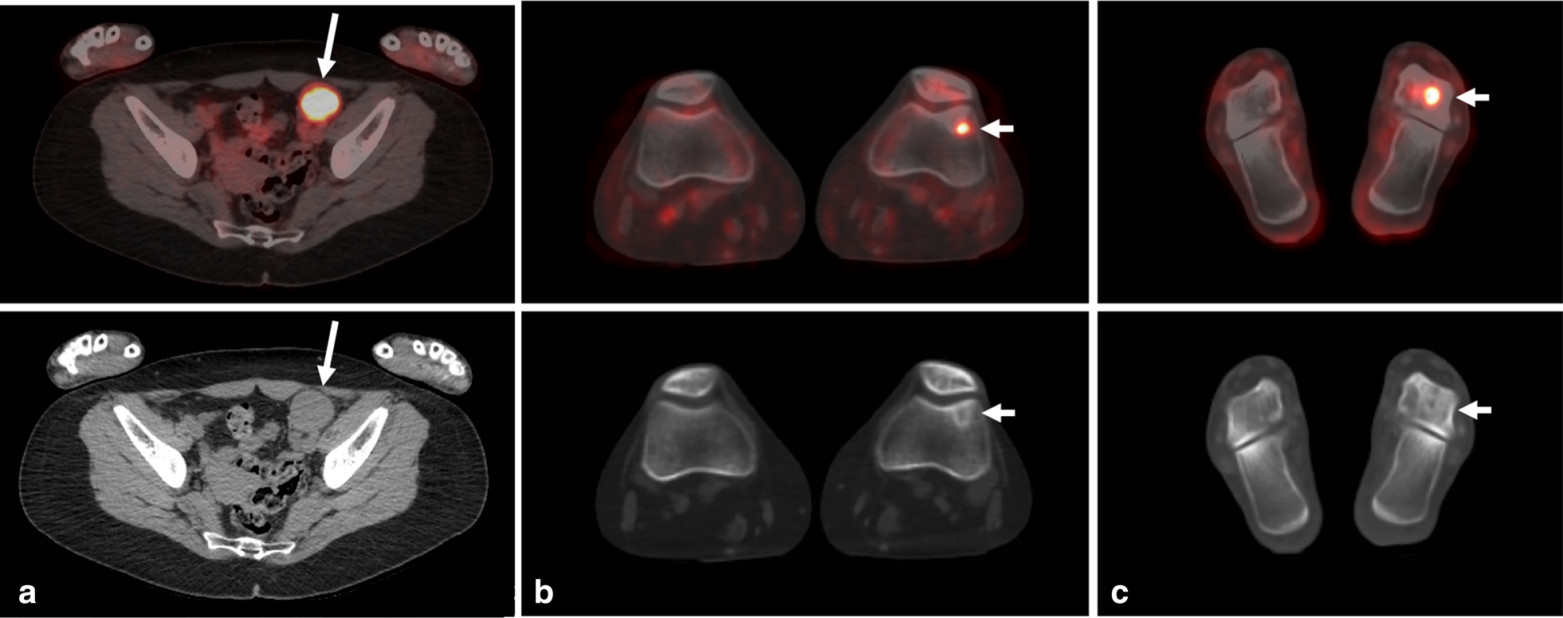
Whole-body ^18^F-FDG PET/CT demonstrates pelvic lymphadenopathy and osseous lesions. a Intense uptake in a 3.8 × 3.6 cm left pelvic side wall lymph node on the fused PET/CT axial image (SUV_max_ 9.8, white arrow). b Moderate FDG uptake in the left lateral femoral condyle (SUV_max_ 3.1, white arrow), on the fused PET/CT axial image, correlating with the mixed lytic/sclerotic subchondral lesion in the CT. c Intense FDG uptake in the left talus on the fused PET/CT axial image (SUV_max_ 7.7, white arrow) correlating with mixed lytic/sclerotic subchondral lesion on the CT
